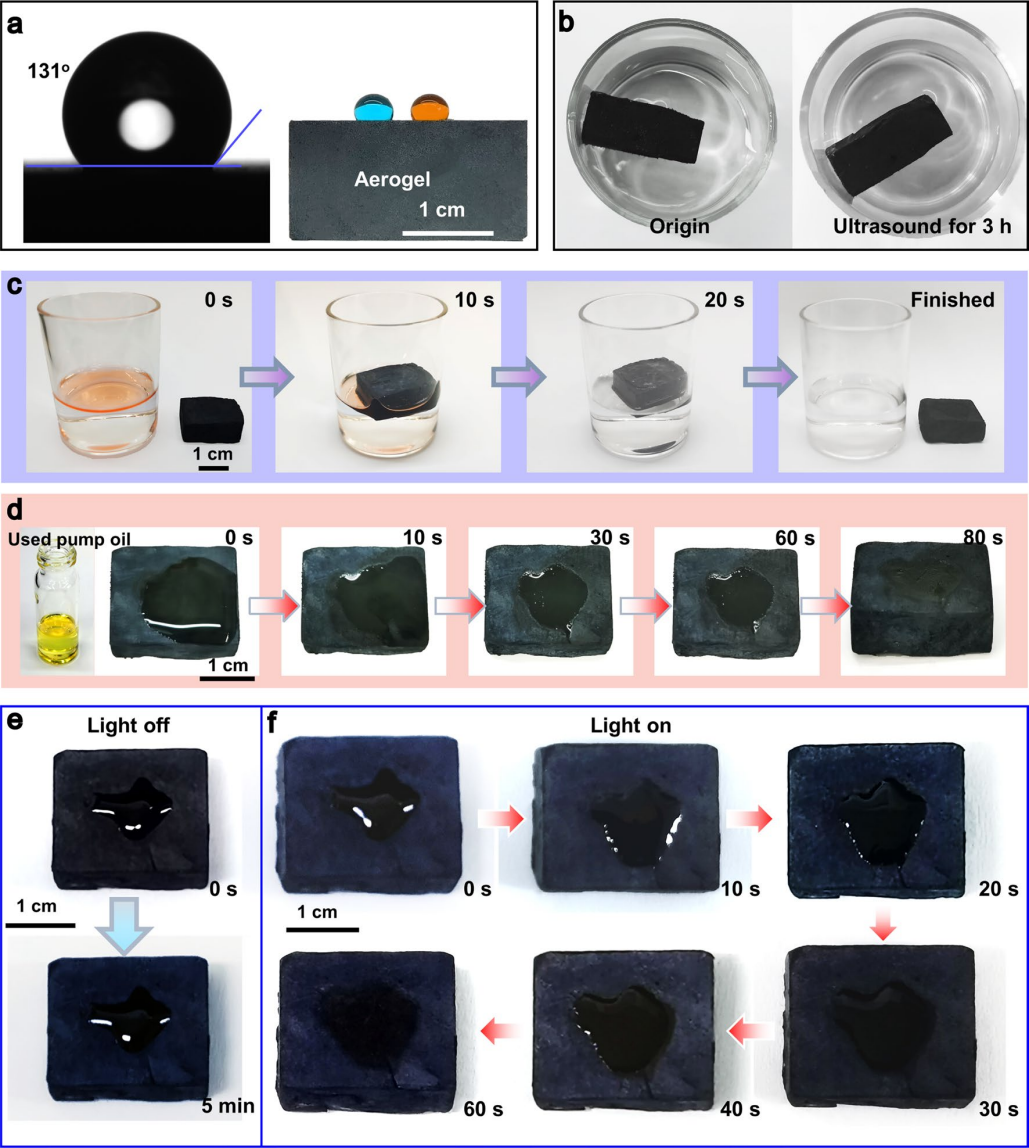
a Water contact angles of water on the MXene aerogel side of the MG-MXene aerogel. b Digital photographs of MG-MXene aerogel before and after ultrasonic treatment in water for 3 h. c Digital photographs of MG-MXene aerogel absorbing an organic solvent. d Absorption of used pump oil. Adsorption of crude oil e without and f under solar-thermal irradiation
